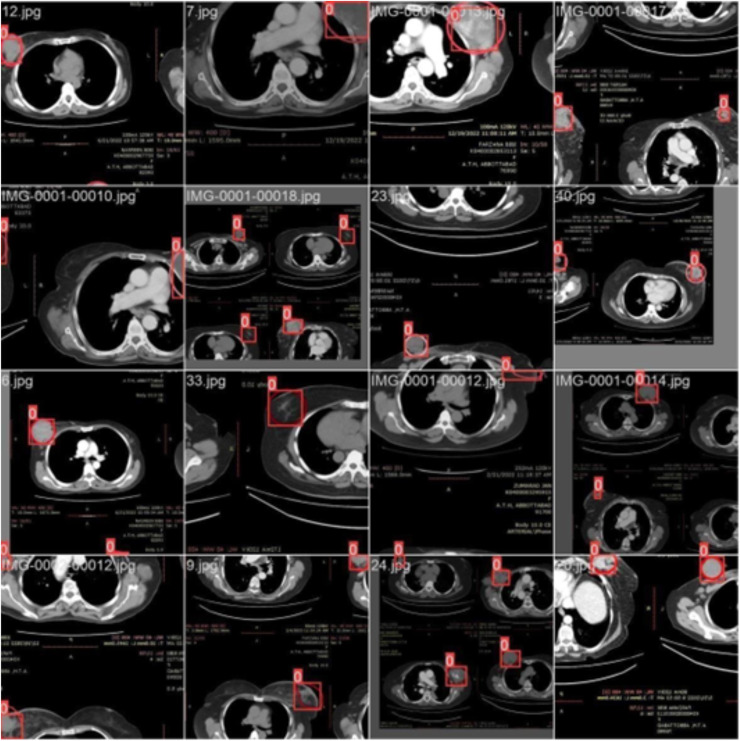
CT scan of breast cancer of 16 diseased patients with training samples in batch 1.
